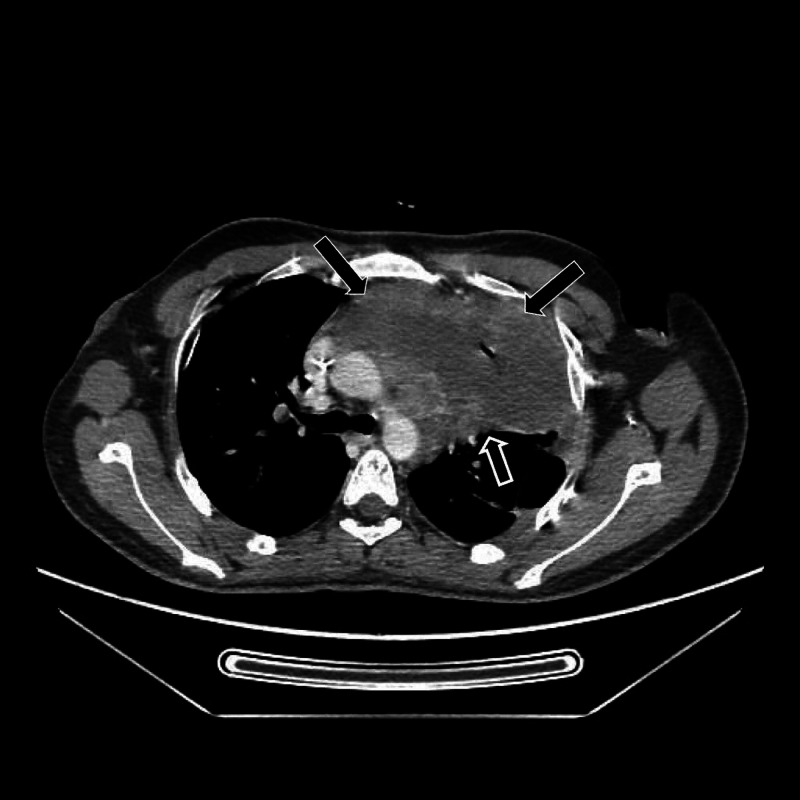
Computed tomography (CT) of the chest showing a large mass in the superior mediastinum.
